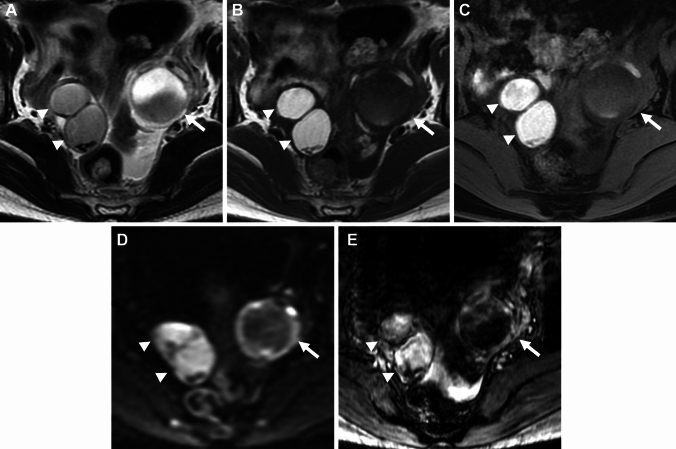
Endometrioma (typical) and hemorrhagic corpus luteum cyst. A 40-year-old with a history of pelvic pain and suspicion of bilateral ovarian cysts on ultrasonography examination. A T2-weighted image reveals bilateral ovarian cysts. The right cysts (arrowheads) show a faint, layered signal loss, whereas the left cyst (arrow) shows a partial signal loss. T2 dark spots are demonstrated in the right posterior cyst. B On T1-weighted image, the right cysts show high signal intensity (≥ fat) and the left cyst exhibits low signal intensity. No signal decrease is observed in the right ovarian cysts on C fat-saturated T1-weighted image. Multiple T1-high signal intensity cysts (multiplicity), the shading sign (T2-shortening in adnexal cyst exhibiting T1-high signal intensity), and the presence of T2 dark spot suggest right ovarian endometriomas. The left cyst is suggestive of the non-endometriotic hemorrhagic cyst (corpus luteum cyst). D Diffusion-weighted image (DWI) (*b* = 800 s/mm^2^) shows totally high signal intensity in the right cysts, and ring-like high signal intensity in the left cyst. E Susceptibility-weighted image (SWAN: susceptibility-weighted angiography) reveals dotty to curved linear signal voids in the walls of the right cysts. Signal voids are prominent within the left cysts but not in the cyst wall
